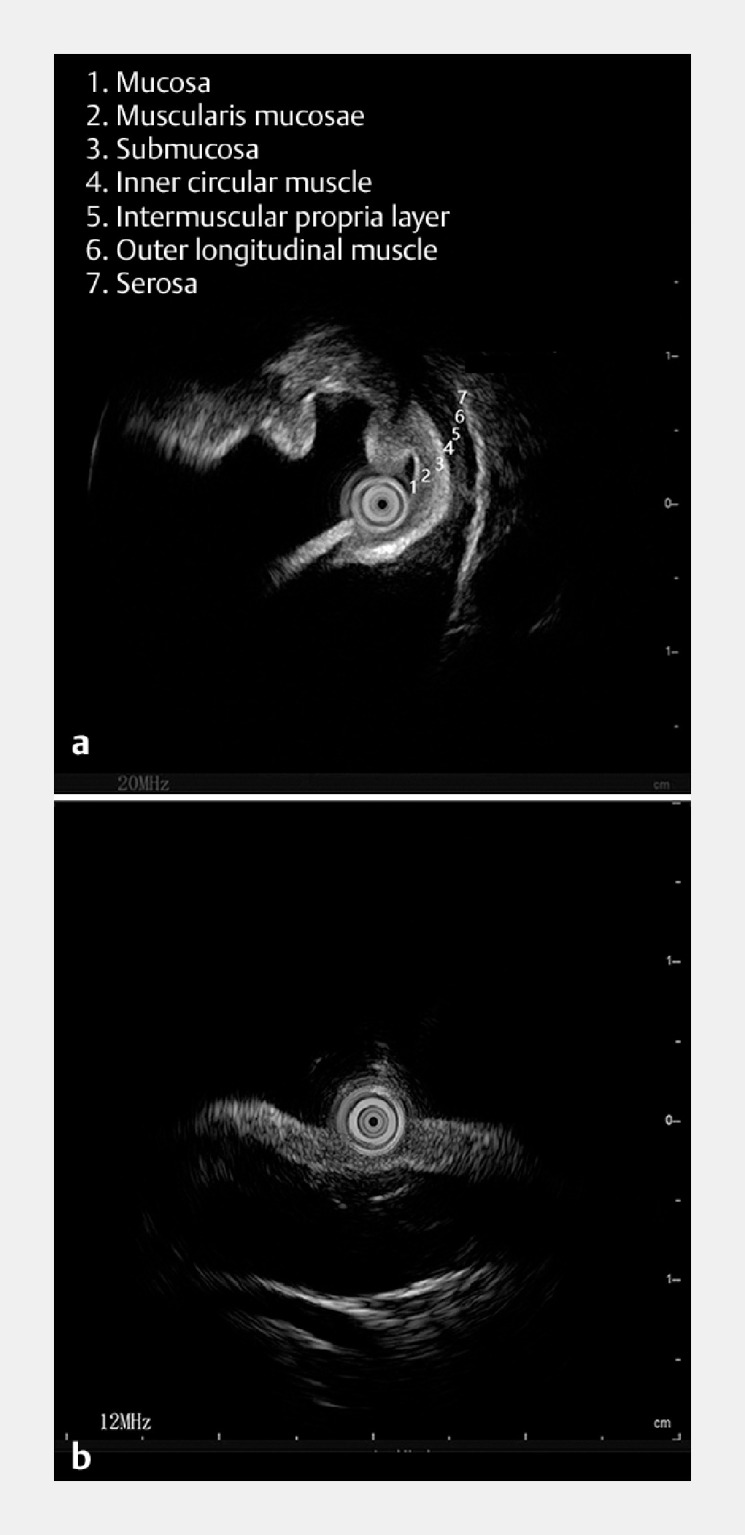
Utilization of the dual-frequency ultrasonic miniprobe in the diagnosis of giant gastric subepithelial lesions.
